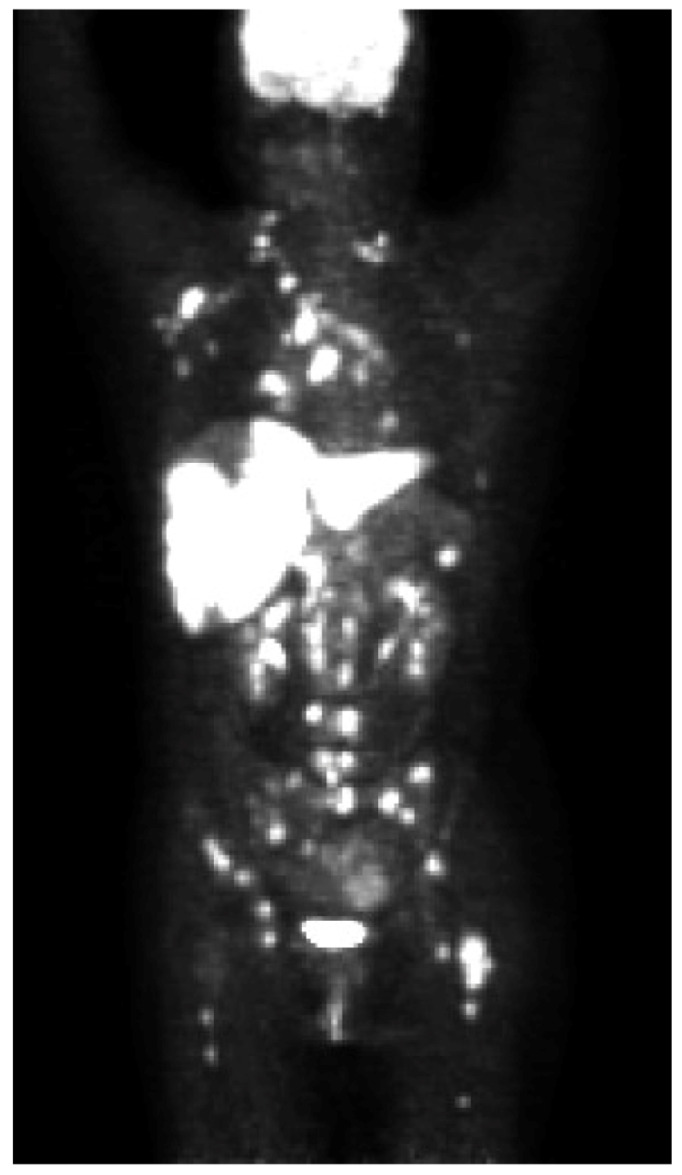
FDG-PET/CT performed before treatment. The figure shows FDG uptake in multiple lesions (liver, bone, and lymph node lesions). FDG-PET/CT: fluorine-18-2-deoxy-d-glucose positron emission tomography and computed tomography.
